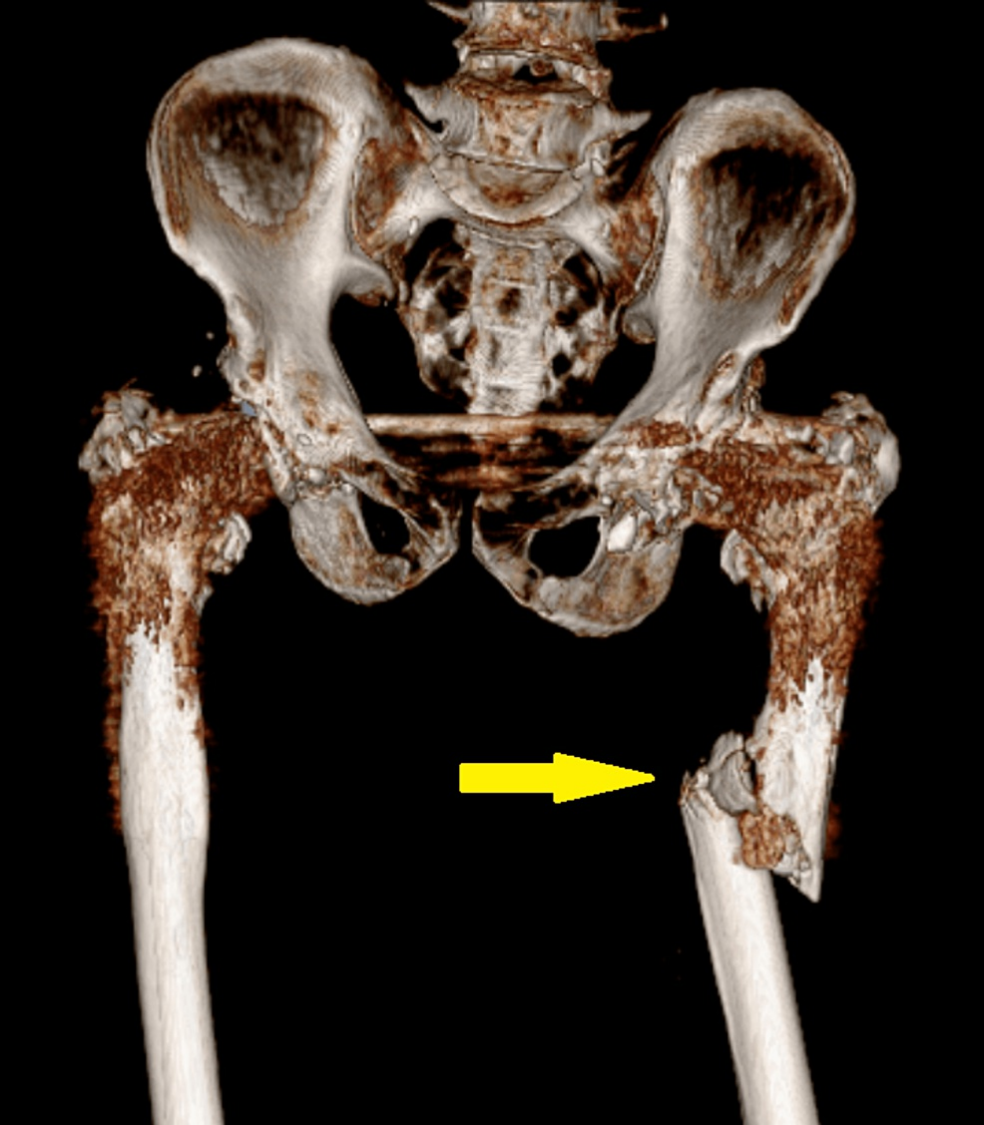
Pre-operative 3D reconstruction computed tomography scan of pelvis with both hip joints shows periprosthetic femur fracture left side
